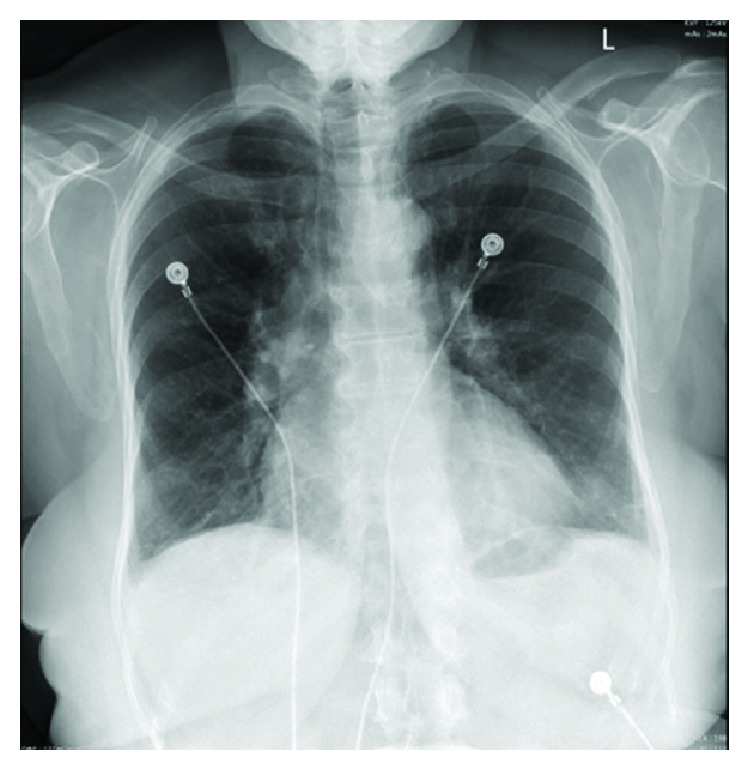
Chest X-ray showing cardiomegaly and pulmonary vascular prominence.
